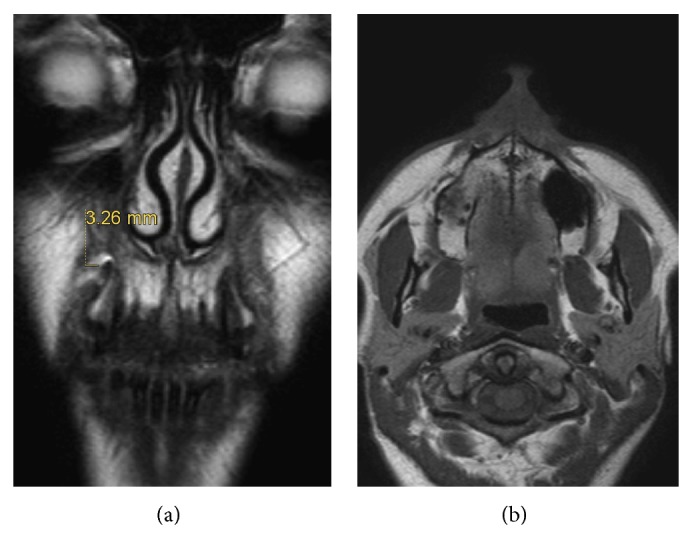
(a)-(b) MRI of the facial skeleton after 3 months from surgery. We can appreciate soft tissue volume deposition in the area of previous fat grafting.
